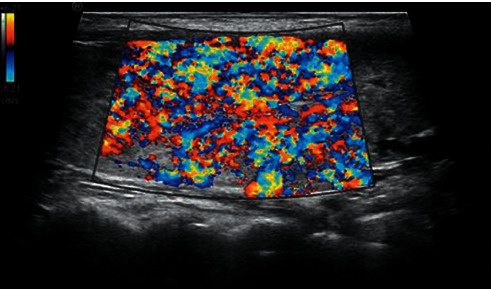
Color Doppler ultrasound grading of hyperthyroidism level 2.
